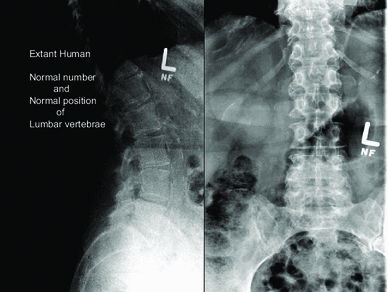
Extant human lumbar X-rays which show the normal number (5) and position of the lumber vertebrae
